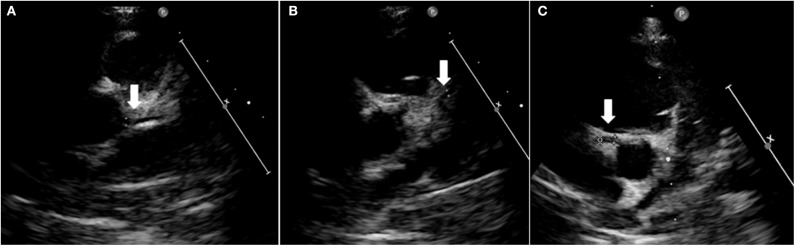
Heart ultrasound showing normal coronary arteries at 1-year follow-up. Left main coronary artery (A), left anterior descending artery (B), and right coronary artery aneurism (C).
